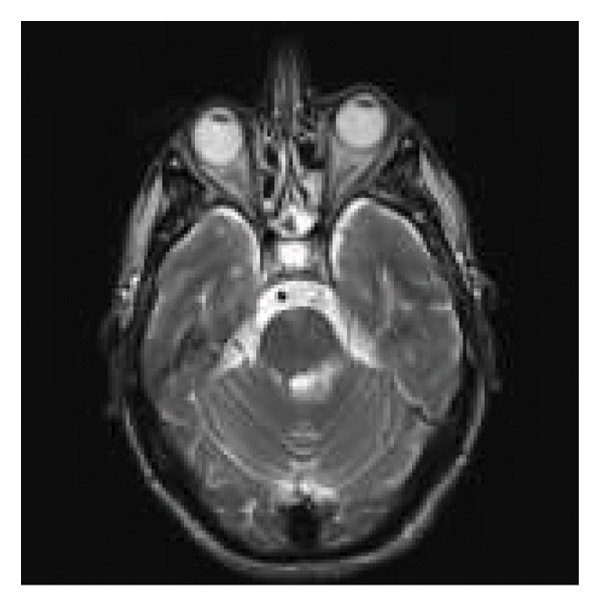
Axial brain MRI, FLAIR sequence, showing several hyperintense lesions, the largest located at the left cerebellar peduncle.
